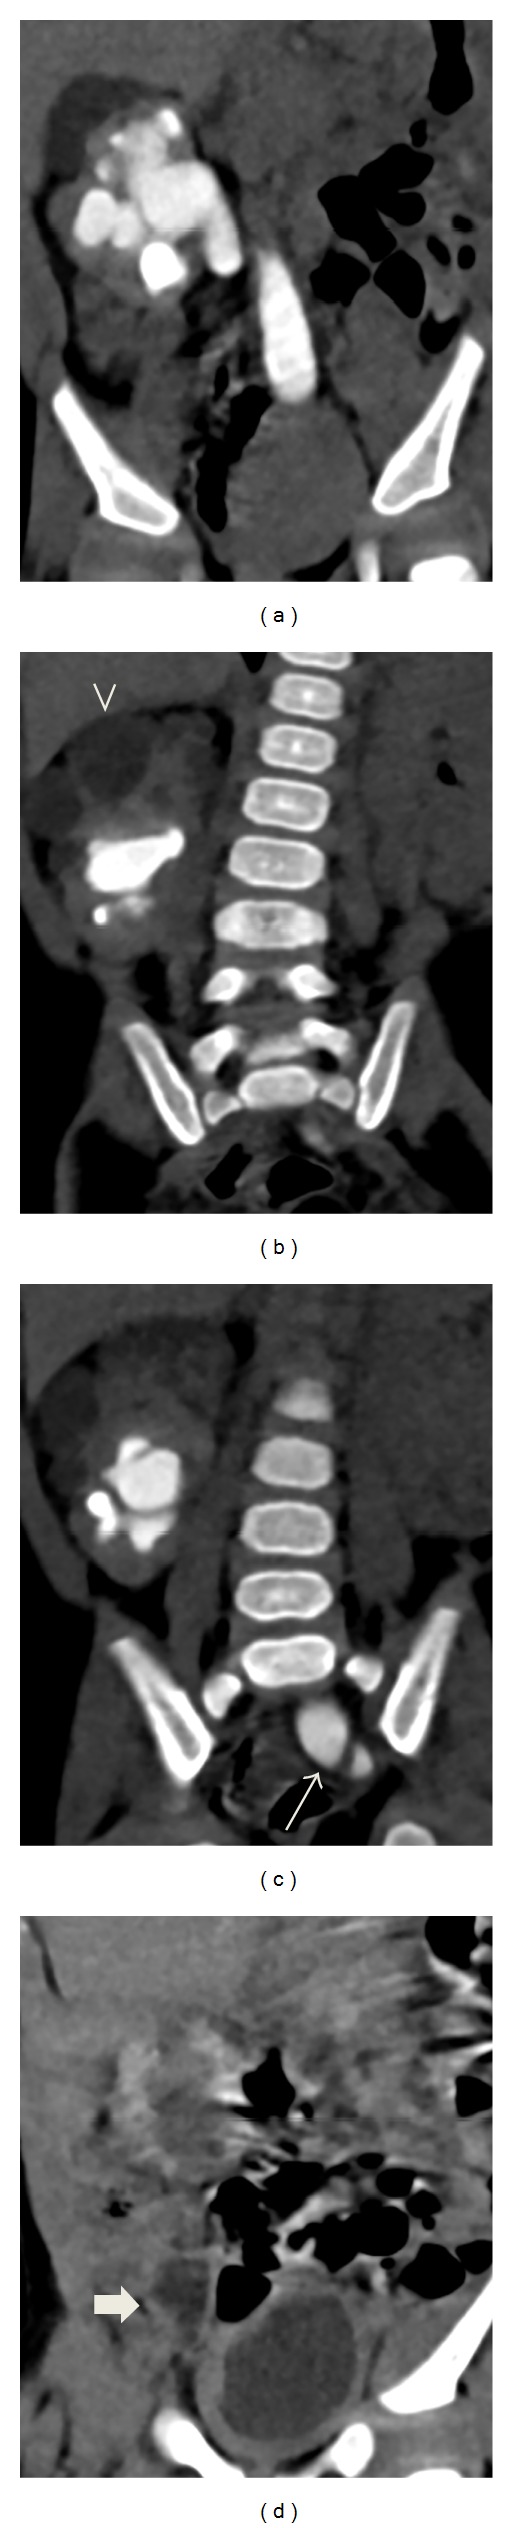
Contrast-enhanced abdomen CT, 6 months of age. (a) moderate hydronephrosis in the left crossed fused renal ectopic kidney; (b) noncommunicating cysts representing the orthotopic right multicystic dysplastic kidney in the superior aspect (arrowhead); (c) dilated tortuous left ureter at the left ureterovesical junction (arrow); (d) nonopacified right distal atretic ureter is not opacified (block arrow) confirming the absence of communication with the nonfunctioning orthotopic right multicystic dysplastic kidney.
